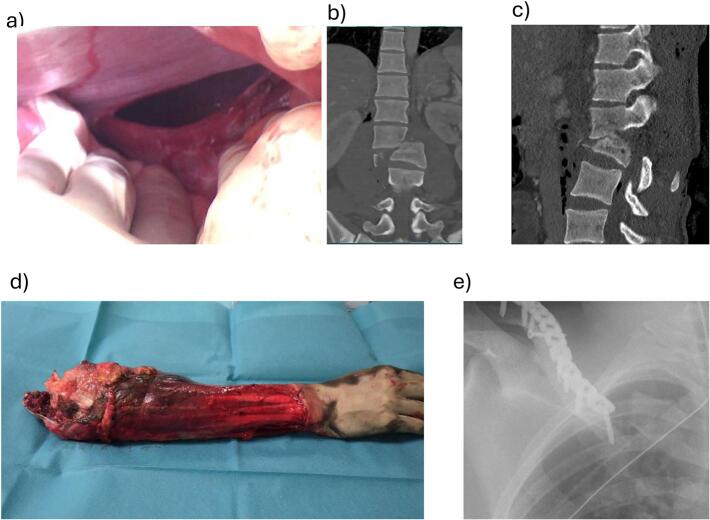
Trauma sequela: a) Diaphragmatic rupture visible in laparotomy, b–c) CT-scan of spinal fracture, d) amputate at occurrence in trauma bay, e) X-ray of peri-implant clavicle fracture.
